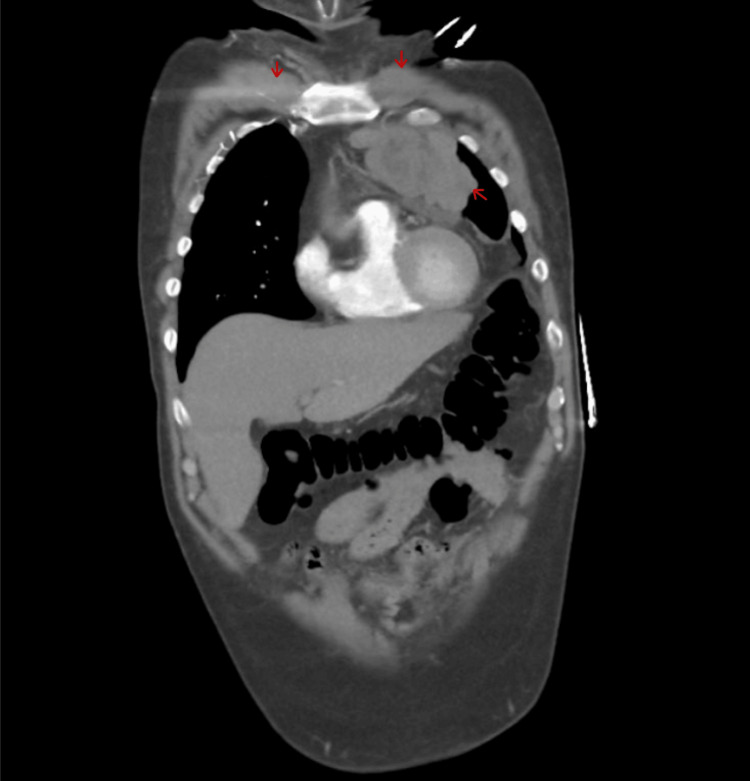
CT chest coronal view shows a mediastinal mass and extensive bilateral supraclavicular lymphadenopathy
